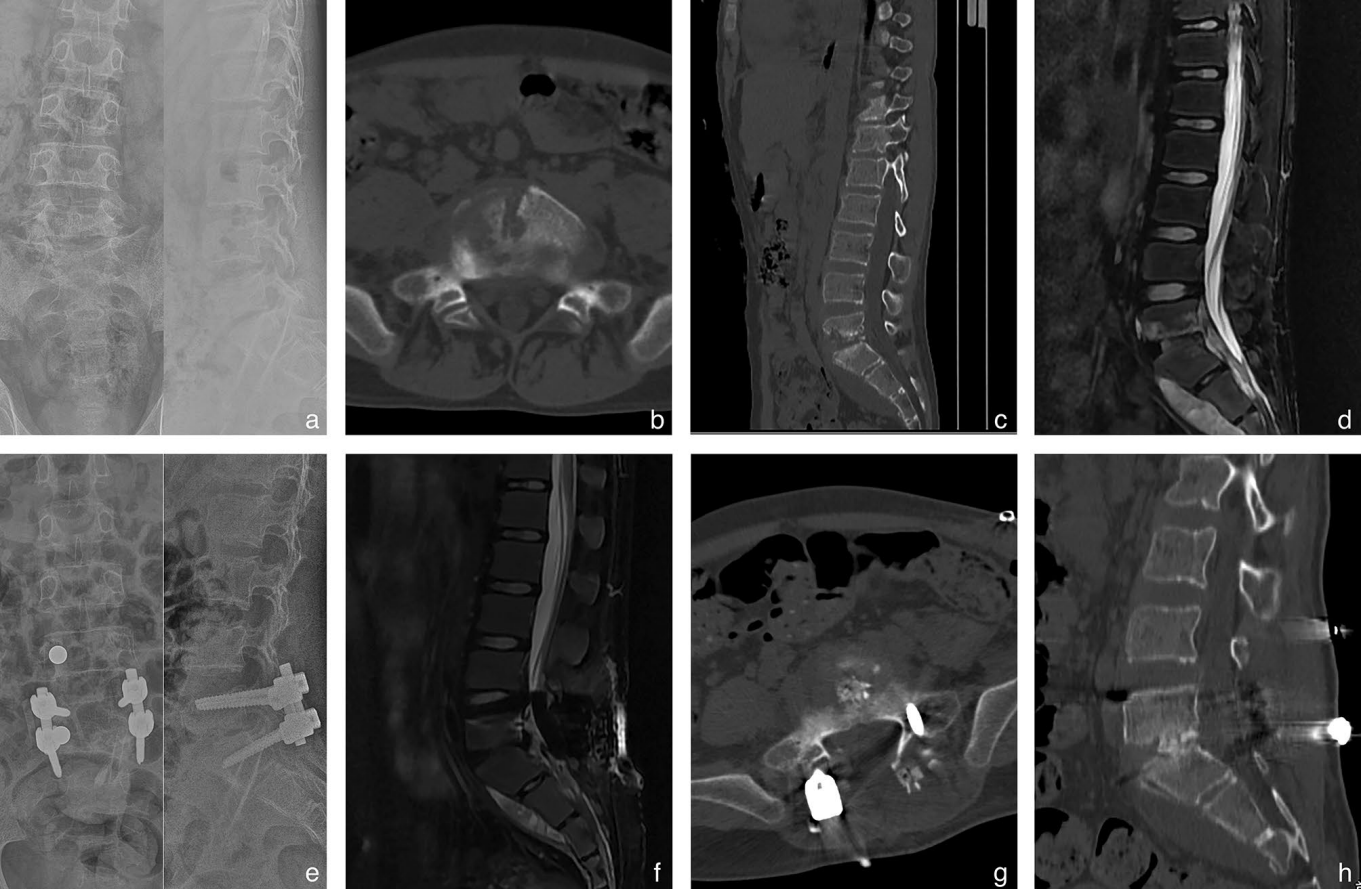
A 22-year-old female patient diagnosed with L5-S1 brucella spondylitis underwent a comprehensive treatment approach consisting of posterior pedicle fixation combined with anterior retroperitoneal debridement and interbody fusion. Preoperative anteroposterior and lateral X-ray, CT, and MRI scans revealed intervertebral stenosis, as well as significant disc and vertebral body destruction. (a–d) The postoperative imaging materials exhibited successful resolution of the infection and confirmed solid bony fusion during the final follow-up (e–h).
